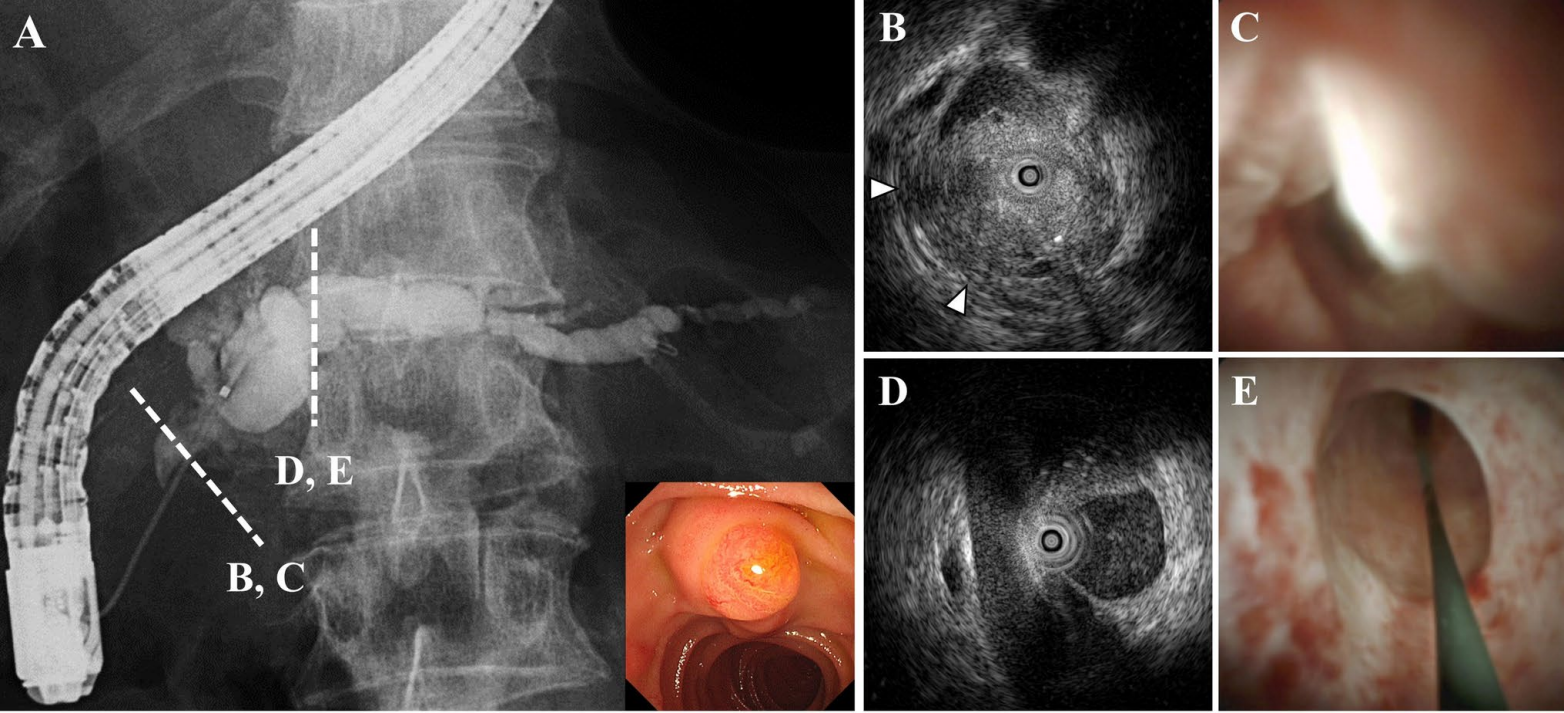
Endoscopic retrograde cholangiopancreatography (ERCP), intraductal ultrasonography (IDUS), and peroral pancreatoscopy (POPS, SpyScope DSII; Boston Scientific, Massachusetts, USA) findings. A ERCP revealed a defect in the main pancreatic duct at the head of the pancreas and dilation of the tail-side main pancreatic duct. B, C IDUS and POPS revealed an intraductal tumor filling the main pancreatic duct and invasion into the pancreatic parenchyma was suspected (arrowhead). D, E The main pancreatic duct in the body of the pancreas was intact, and no masses were observed
